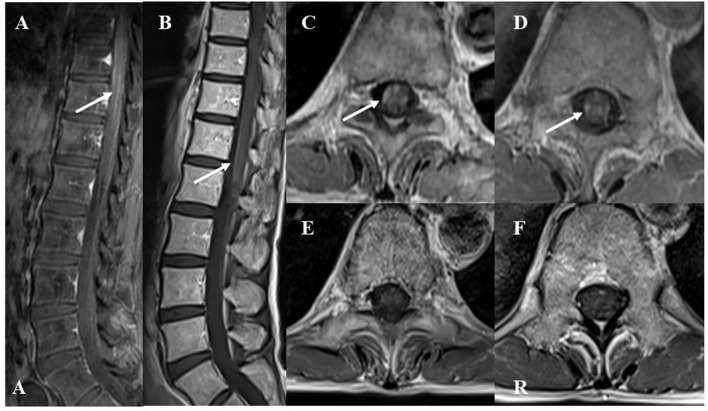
Gadolinium-enhanced spinal T1-weighted magnetic resonance imaging (MRI) findings at recurrence. The lower “A” represents “anterior” in (A). The “R” represents “right” in (F). (A) Sagittal MRI showed abnormal enhanced lesions in the epiconus to cauda equina. (B) After two courses of high-dose steroid pulse therapy, follow-up MRI showed decreased enhancement in the epiconus to cauda equina at recurrence. (C,D) Axial images showed abnormal enhanced lesions at the 9 and 12th levels of the thoracic spine. (E,F) After two courses of high-dose steroid pulse therapy, follow-up MRI showed decreased enhancement at the 9 and 12th levels of the thoracic spine.
